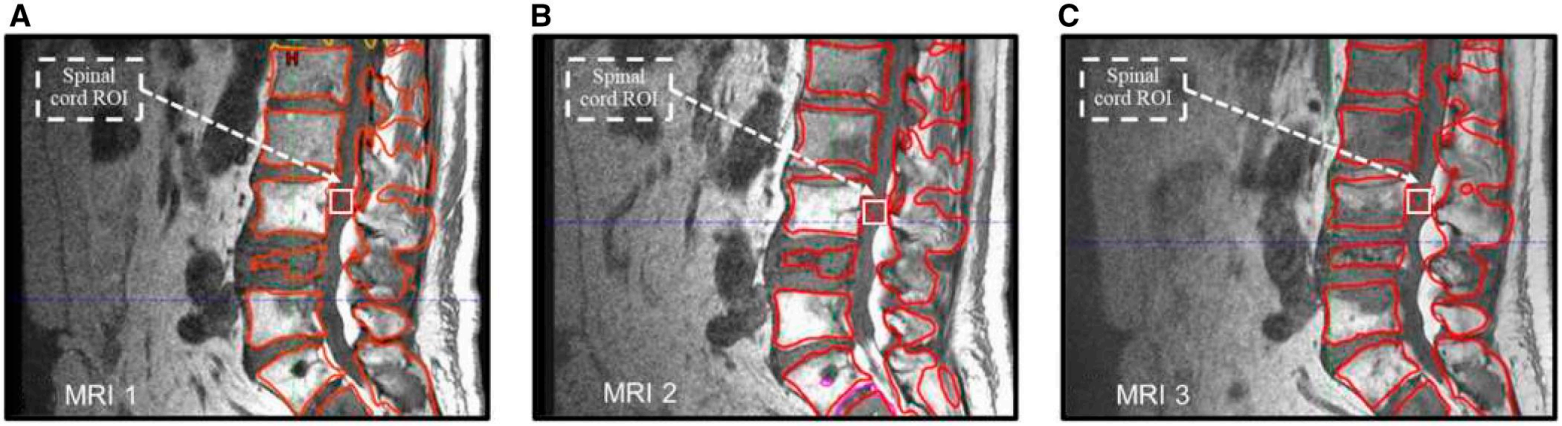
T1w MRI contours of the vertebrae outside the EBRT field for (A) MRI1, (B) MRI2, and (C) MRI3. The white square represents the spinal cord ROI of 1 × 1 cm^2^ chosen as reference for the normalization.
